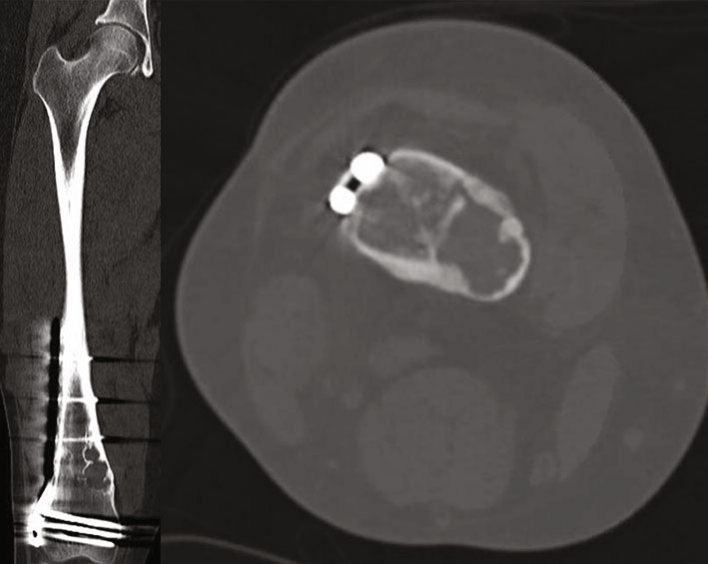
Computed tomography (CT) obtained 9 months status postopen reduction internal fixation of the distal femur showing a healed fracture without any evidence of any intra-articular hardware penetration.
